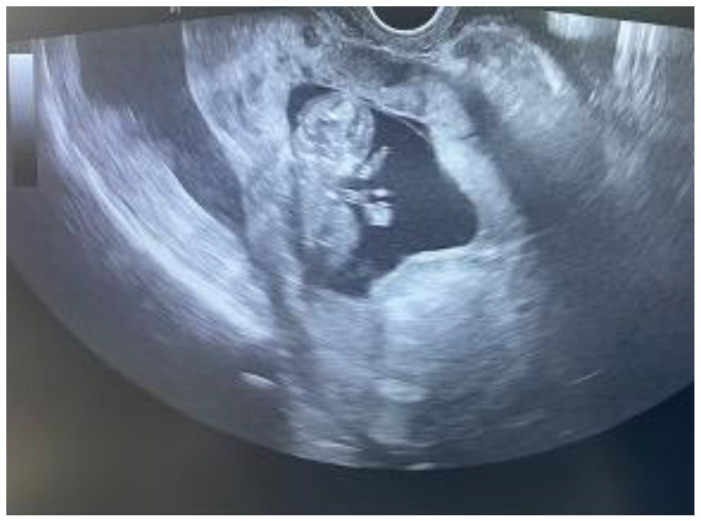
Transvaginal ultrasound scan.
